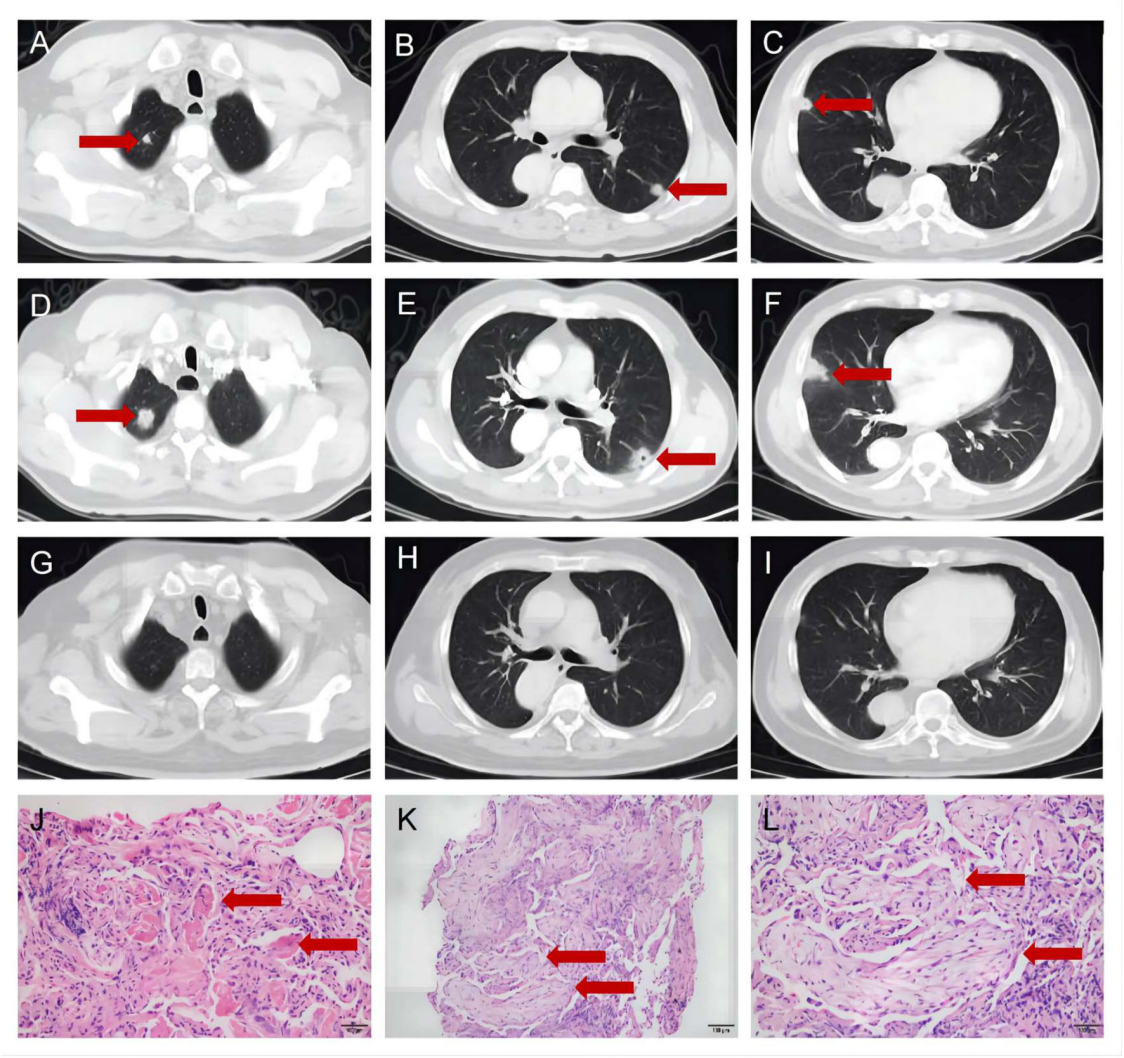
The chest high-resolution computed tomography and pathology of bronchoscopy biopsy. (A–C) The chest high-resolution computed tomography at onset indicated irregular nodular opacities in the upper and lower lobe of the right lung and the middle lobe of left lung (Red arrows). (D–F) The chest high-resolution computed tomography after 1-week antibiotic therapy indicated expanded lesions (Red arrows). (G–I) The chest high-resolution computed tomography at 2-month follow-up showed complete resolution of previous lung lesions (Red arrows). (J–L) The pathology of bronchoscopy biopsy showed intra-alveolar fibroinflammatory buds (Red arrows, J), in the form of Masson's bodies (Red arrows, K, L).
